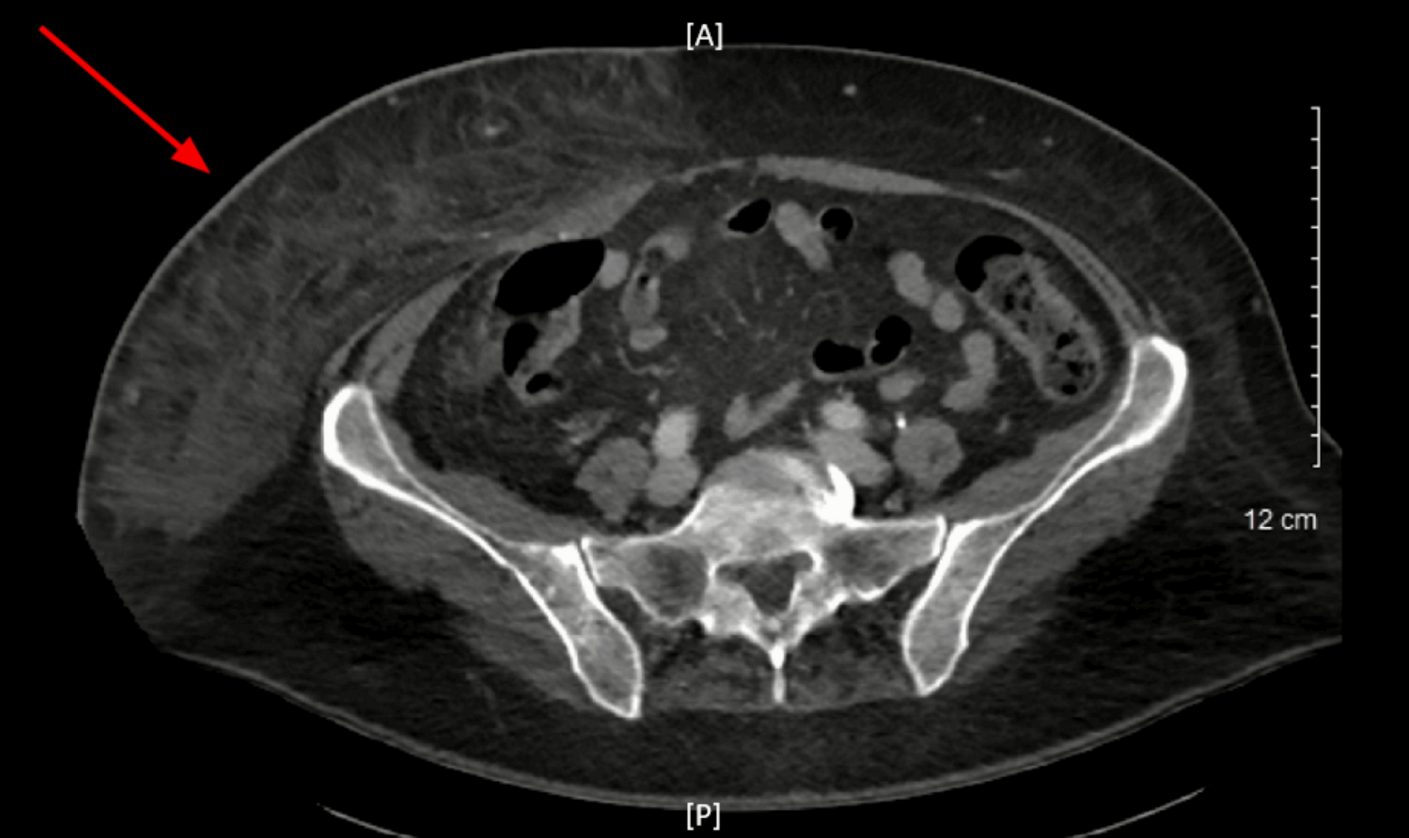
Axial computed tomography scan of the abdomen The red arrow shows skin thickening with extensive subcutaneous fat stranding in the right lower quadrant. These findings correlate to an area of fascial infection assisting with the diagnosis of necrotizing fasciitis. A: anterior; P: posterior
